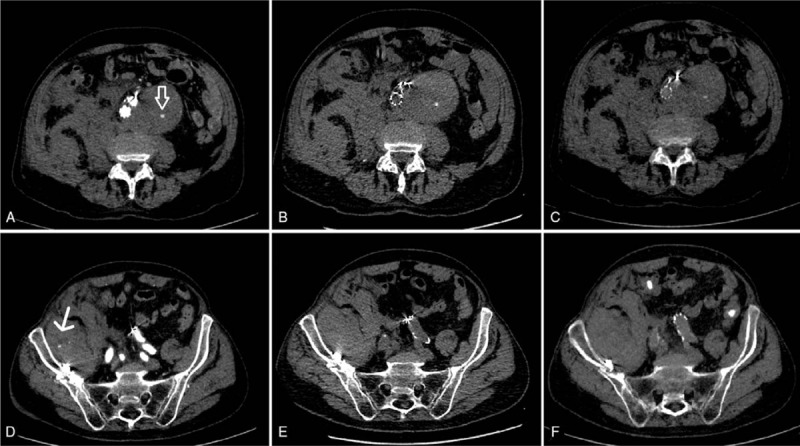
VNC series. 82-year-old male patient who presented to the ER with acute abdominal pain and a drop in Hb from 11.4 to 6.7 g/dL. CT shows a hyperdense dot in an aortic aneurysm (A—arrow) in the arterial contrast phase following EVAR. Both the real unenhanced (B) and virtual unenhanced phase (C) also show the hyperdense dot sign, consistent with calcification within the aneurysm. In this case, it would not have been possible to rule out active bleeding without an unenhanced CT phase. The same patient additionally shows a pelvic hematoma with a hyperdense dot in the arterial contrast phase (D—arrow). Both real unenhanced (E) and virtual unenhanced (F) scans fail to show a hyperdense dot sign, confirming active contrast agent extravasation consistent with active bleeding. CT = computed tomography, EVAR = endovascular aortic repair, VNC = virtual non-contrast.
